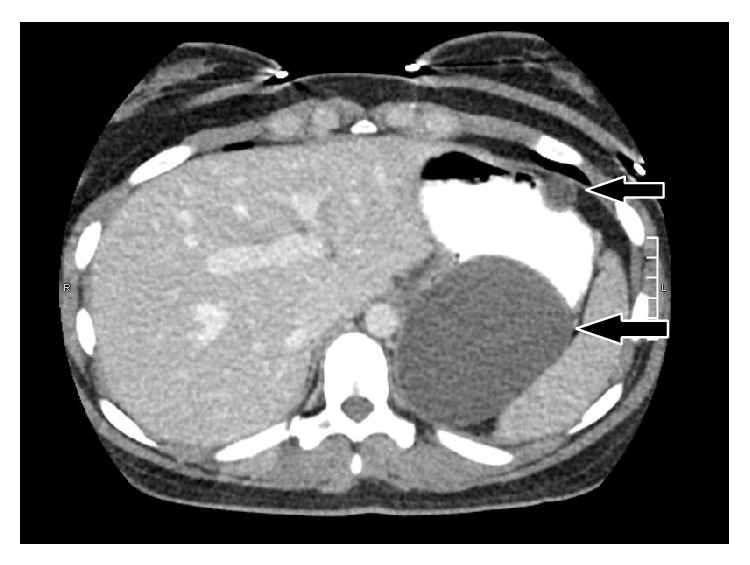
Computed tomography of intra-abdominal cysts. Arrows point to multiple cysts arising from the stomach wall (axial).
